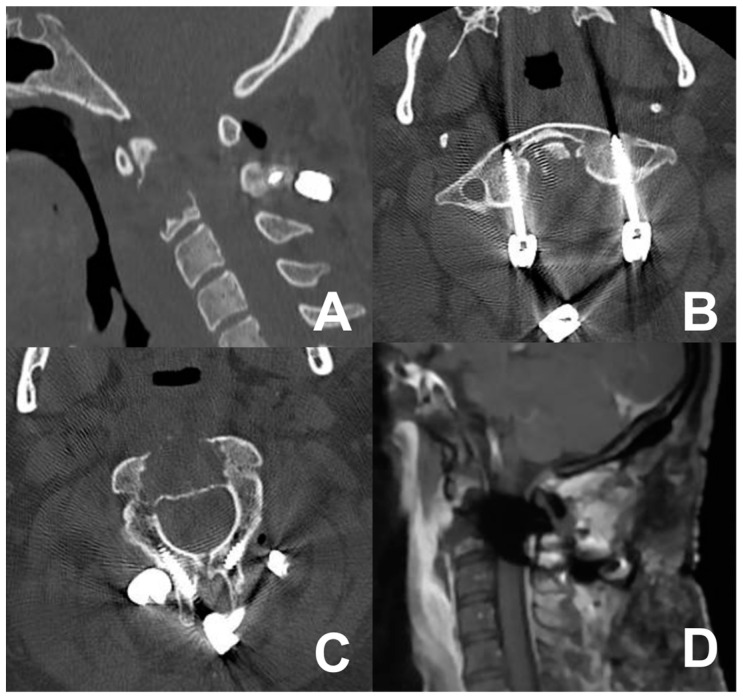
Postoperative CT scan (A–C) and MRI (D) showing the extent of bone removal, tumor resection, and posterior fixation.
